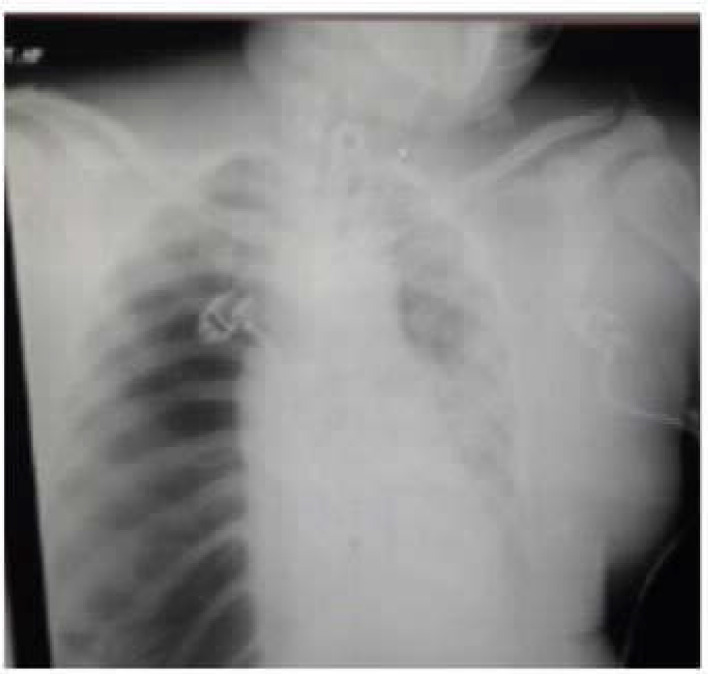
CASE 5: Right Pneumothorax in chest X-ray
